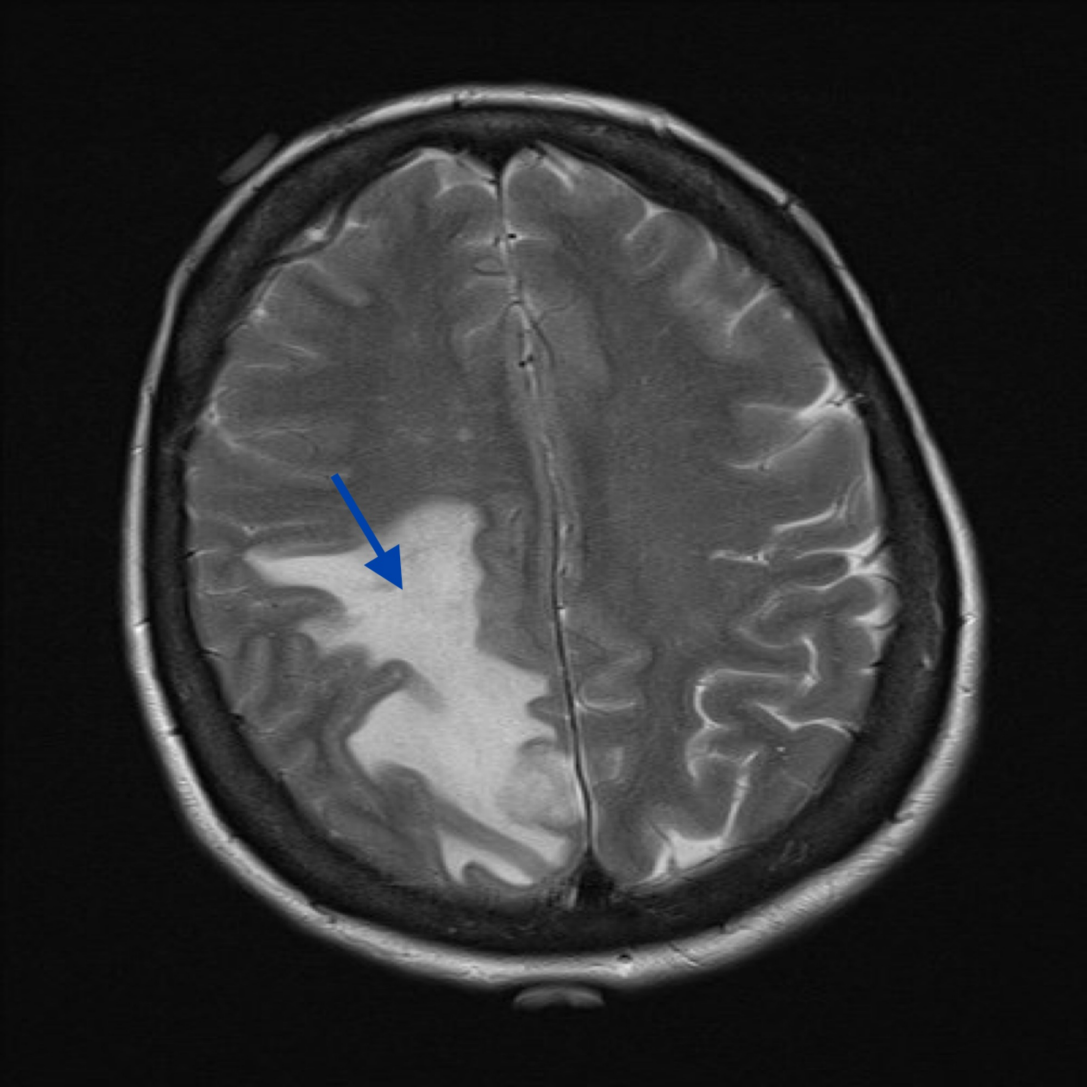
MRI, axial T2 demonstrating vasogenic edema associated with lesion MRI: magnetic resonance imaging
